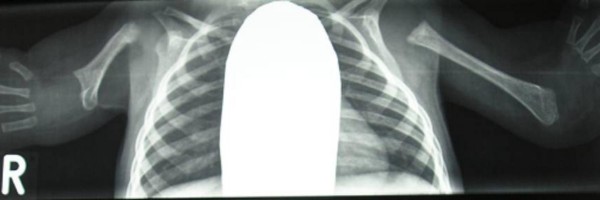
Radiographic examination showed, hypoplastic humeri, agenesis of the radii and ulnae, defective ossification of the carpals and hypoplasia of the distal phalanges.
